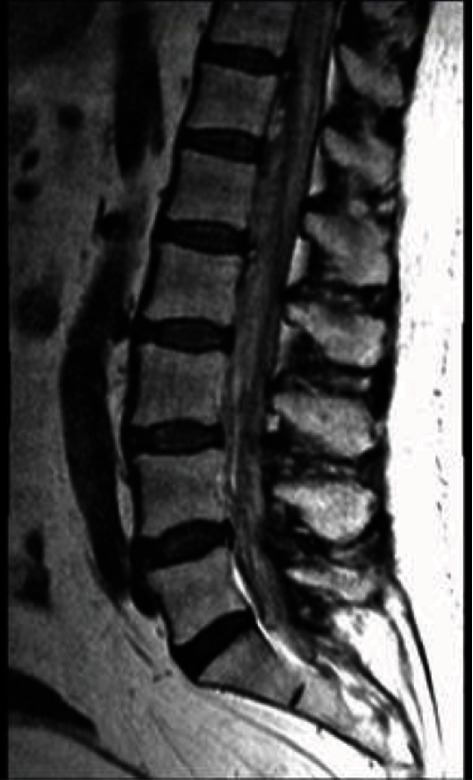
T2-weighted MRI of the lumbar spine demonstrating ventral hypointensity relative to CSF, compressing the conus and cauda equina.
